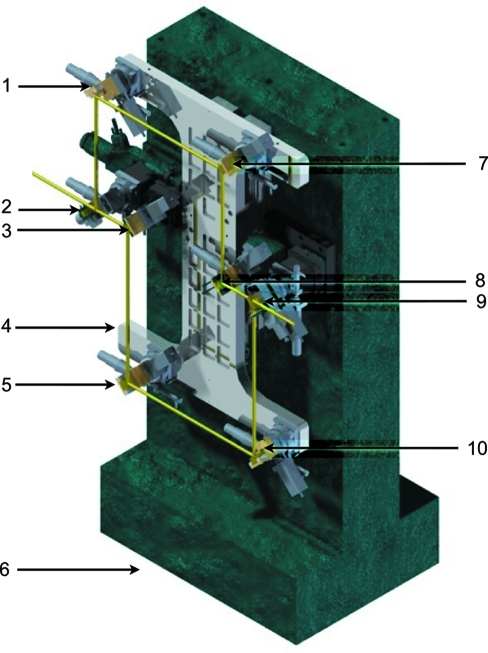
Three-dimensional model of the delay line. (1), (3), (5), (7), (8), (10): Bragg reflector stages. (2): Beam splitter stage. (9): Beam mixer stage. (4): Aluminium plate. (6): Granite support. The X-ray beam path is indicated by the yellow line.
